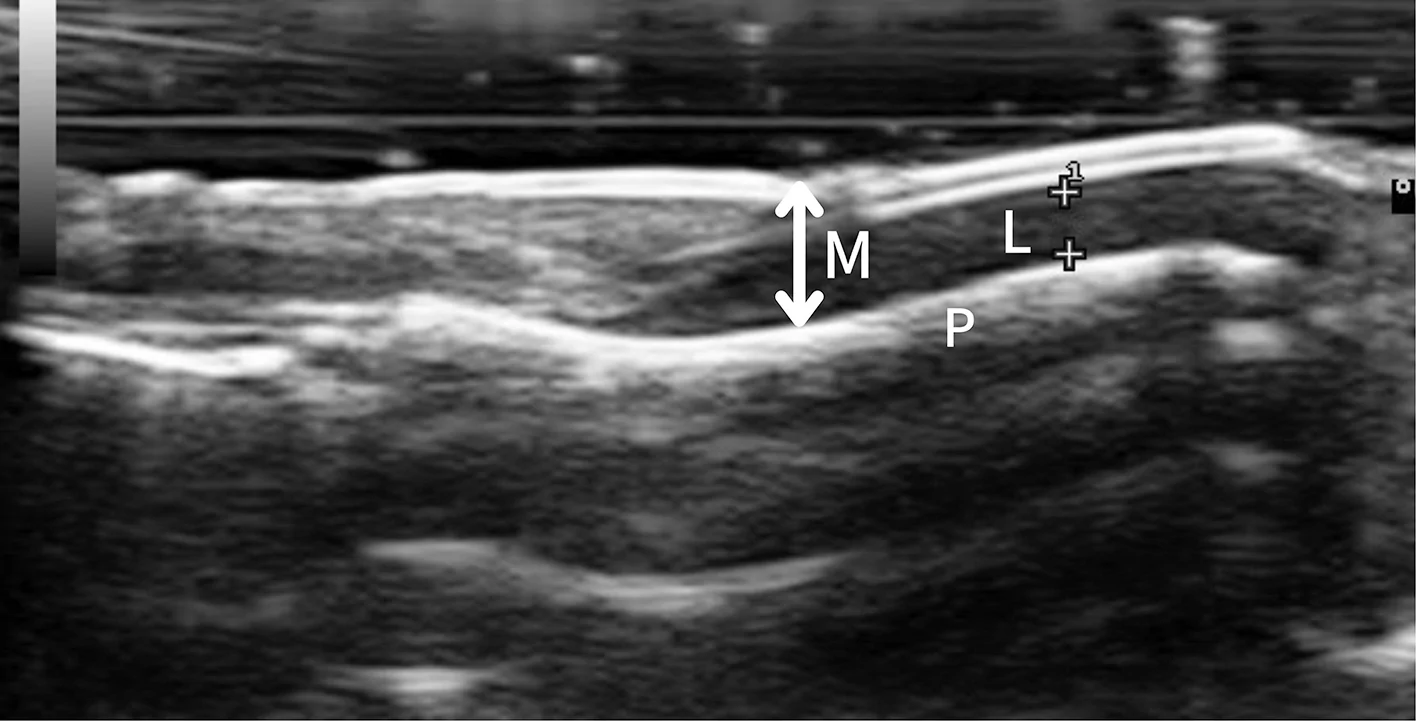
Ultrasound anatomy and nail measurements legend. L- nail bed; M- nail matrix, distance between + is the thickening of the nail bed (between the ventral nail plate: +1 and the periosteum of the distal phalanx: P)
